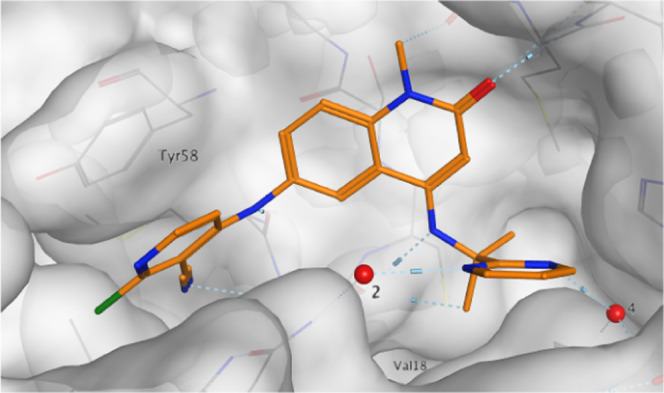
X-ray structure of 13g (PDB: 7OKM, resolution 1.48
